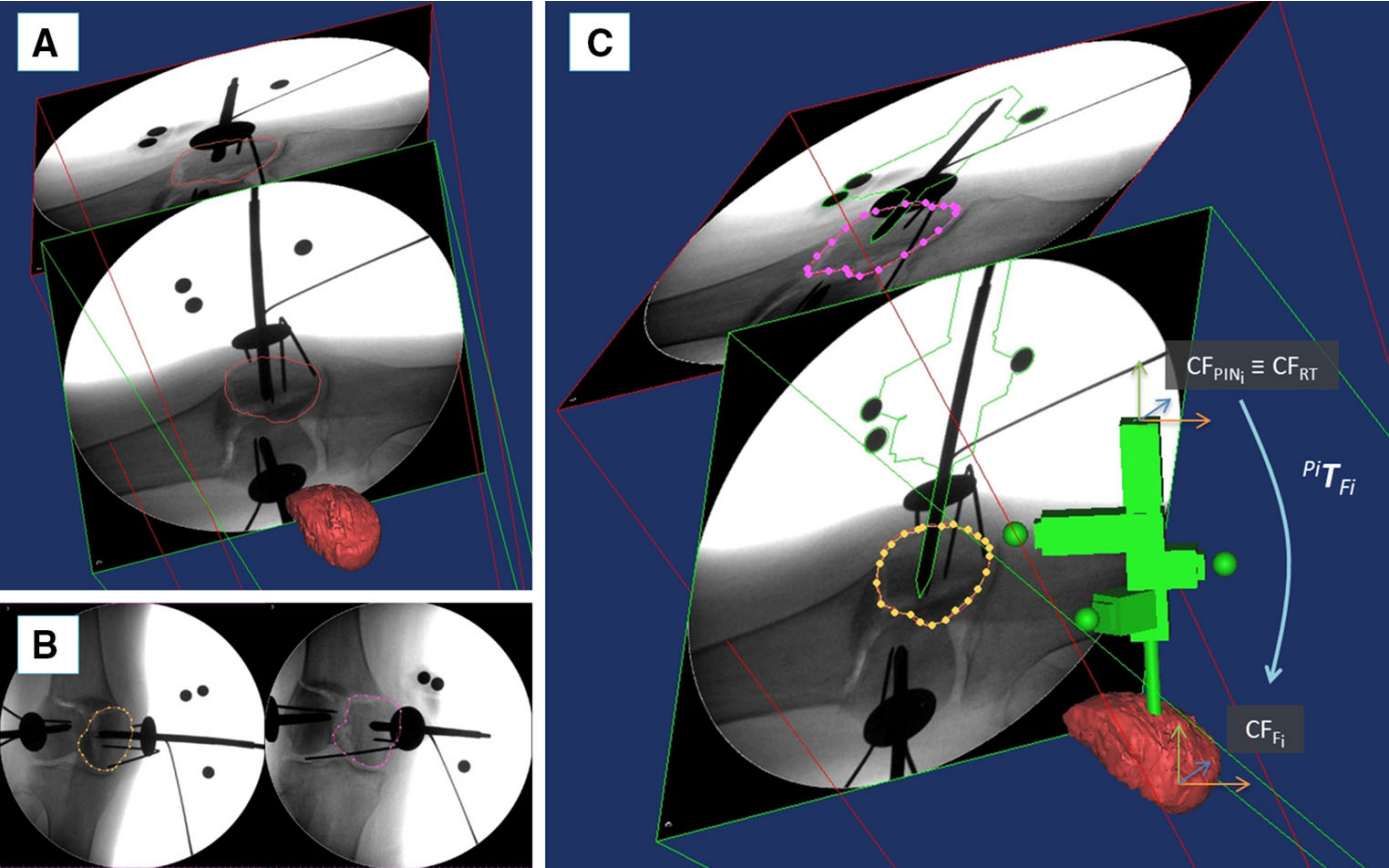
Image registration framework: registration. The CT-generated 3D model of a fractured bone fragment is imported into the reduction software and its contour projected onto the two fluoroscopic images (a). The contour of the corresponding bone fragment is segmented on the fluoroscopic images (b). The 3D model of the bone is registered with the fluoroscopic images, and the relative pose of the pin with respect to the bone is calculated (c)
